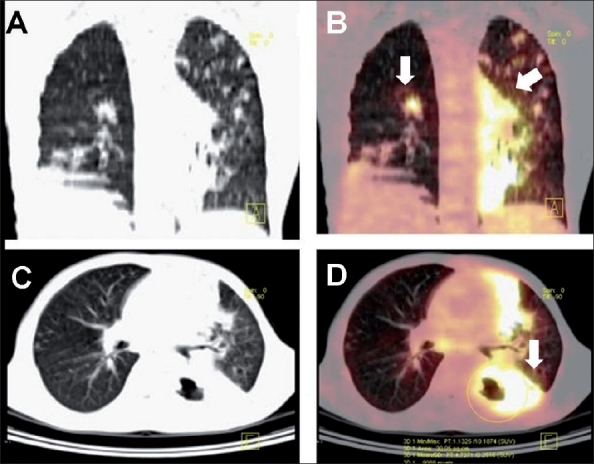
Pulmonary tuberculosis. A sputum positive case of pulmonary TB with clinically poor response to 2 months of antituberculous therapy. Coronal plain CT (A) and PET/CT (B) with axial plain CT (C) and PET/CT (D) images reveal extensive FDG-avid pulmonary parenchymal lesions. The superior segment of the left lower lobe shows consolidation with central cavitation (arrows) with an SUV_max_ of 10.1. These fi ndings suggest active disease, indicating an inadequate response to therapy
